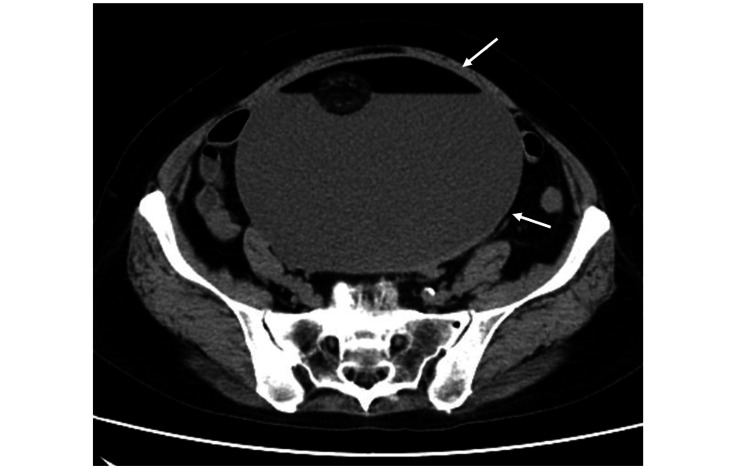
Ovarian tumor on CT Contrast-enhanced abdominal CT revealed the lack of an enhanced component in the tumor (arrows).
